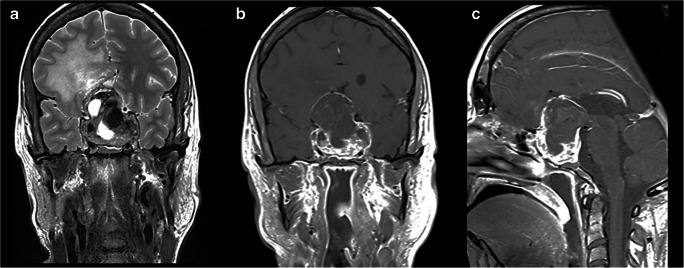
(Patient 2) MR imaging at presentation including (A) coronal T2, (B) post-contrast coronal T1 and (C) post-contrast sagittal T1 sequences documents a large heterogeneous mass lesion with heterogeneously enhancing solid and cystic components and intra-tumoural haemorrhage (A&B). The mass expands the sella and invades into the cavernous sinuses bilaterally. There is marked suprasellar extension and compression of the optic chiasm. The tumour bulges into the anterior aspect of the third ventricle (C) with secondary oedema and high T2 signal in the right frontal lobe (A)
